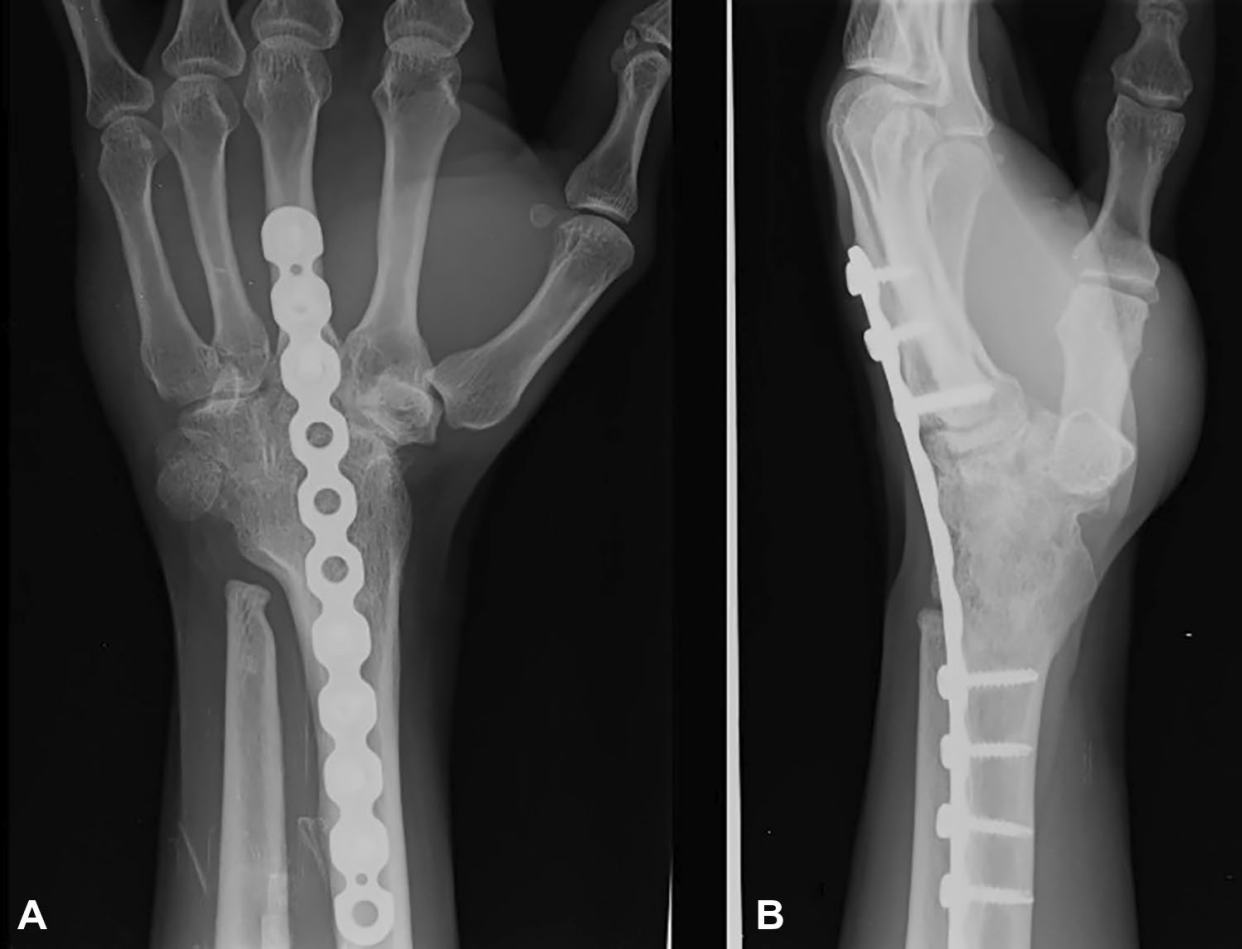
Two years following wrist fusion in a patient with rheumatoid arthritis shows union on radiograph with maintained position and filling up of the donor site
